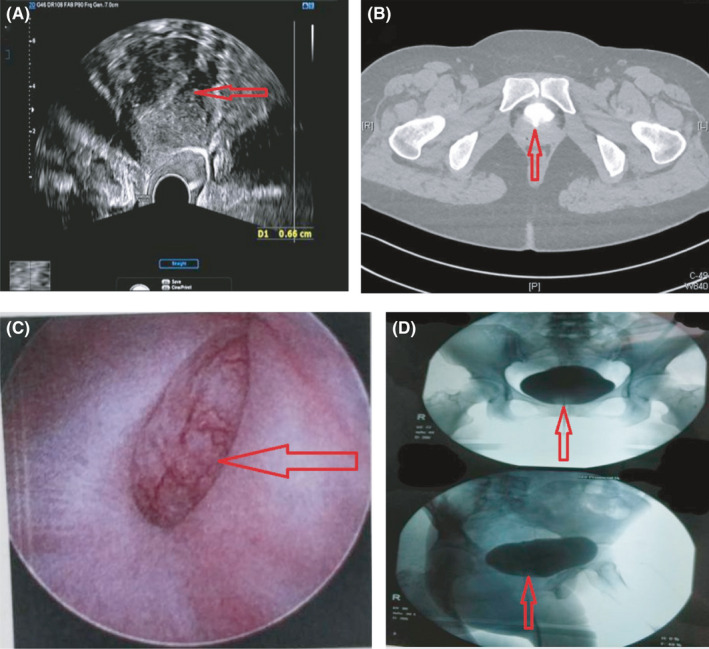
(A) Ultrasound showed that the continuity of the anterior wall of the cervix was interrupted at the level of the internal cervix, the defect was 0.55 cm wide, and the cervical canal communicated with the bladder. (B) Enhanced urography showed speckled high‐density lesions in the uterus below the bladder. (C) Cystoscopy showed that bladder wall defect was visible above the triangle of bladder, with a range of about 3 cm × 2 cm. D Methylene blue +contrast medium bladder instillation test showed slight vaginal leakage
